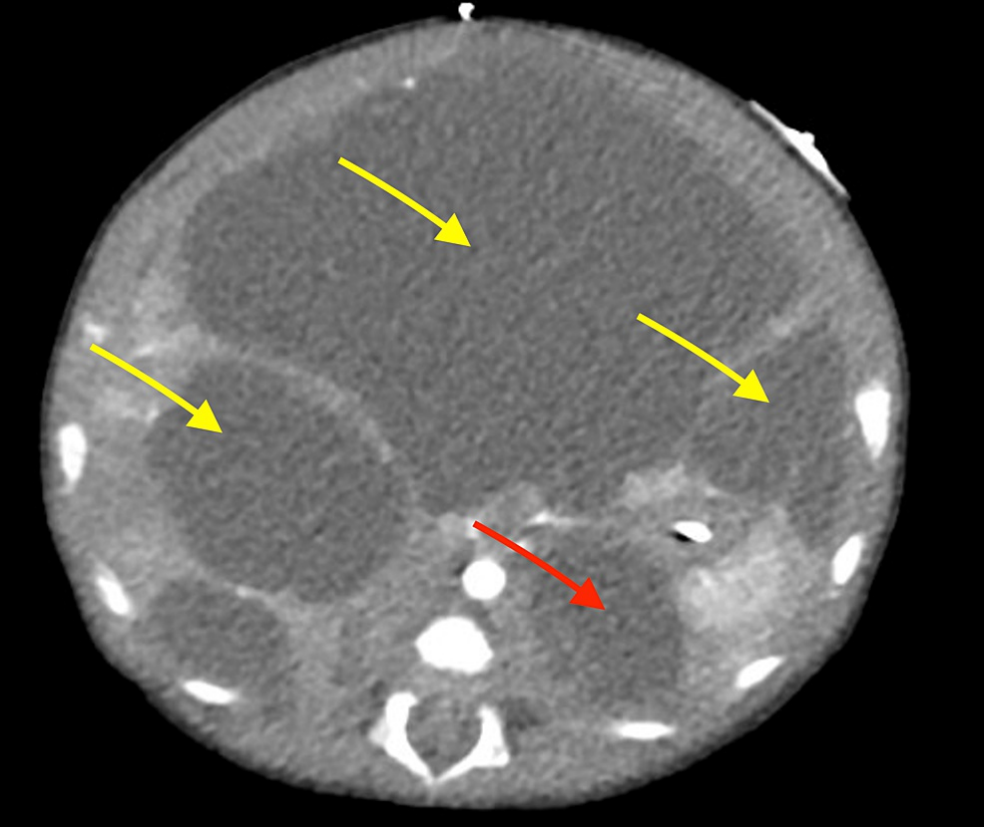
Axial enhanced CT demonstrates large, well-circumscribed, fluid-attenuating cysts in the liver causing mass effect (yellow) There is a cyst seen in the left para-renal/retroperitoneal region (red).
